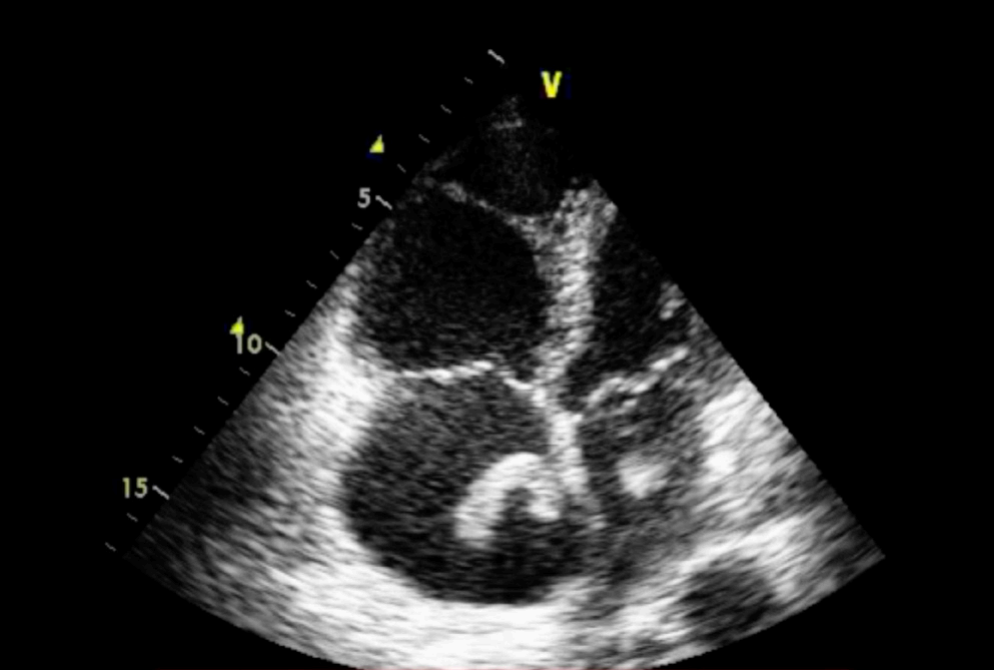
Transthoracic echocardiogram demonstrating right heart dilatation and bi-atrial masses
